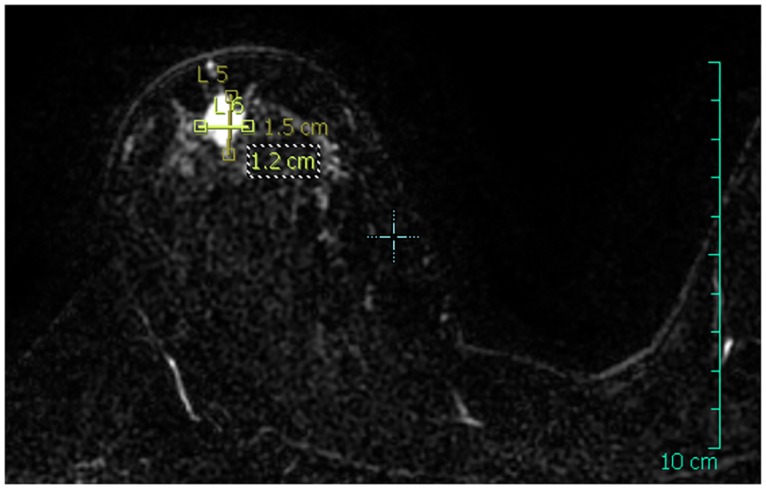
Right breast MRI in the transverse plane. The dimensions of the tumor were measured and annotated on the MRI image.
